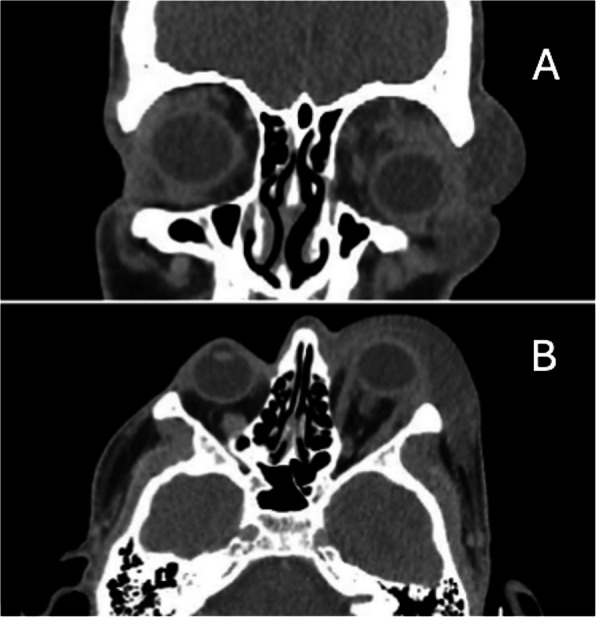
CT scan of patient 3 at presentation showing soft tissue thickening in the superior and lateral aspect of the left orbit, displacing the orbital structures inferiorly, shown in the coronal (A) and axial (B) sections
